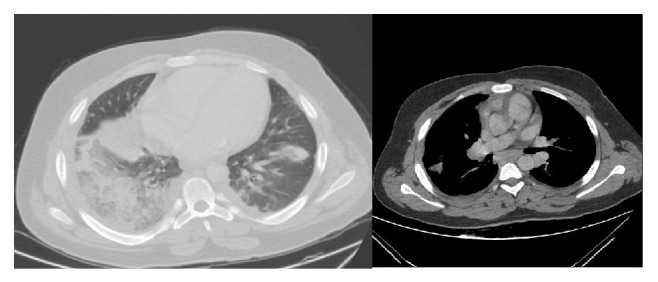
Consolidation areas in the lower lobe of right lung, upper-lower lobe of left lung, and lingula were observed in first drawn contrast enhanced computed tomography.
